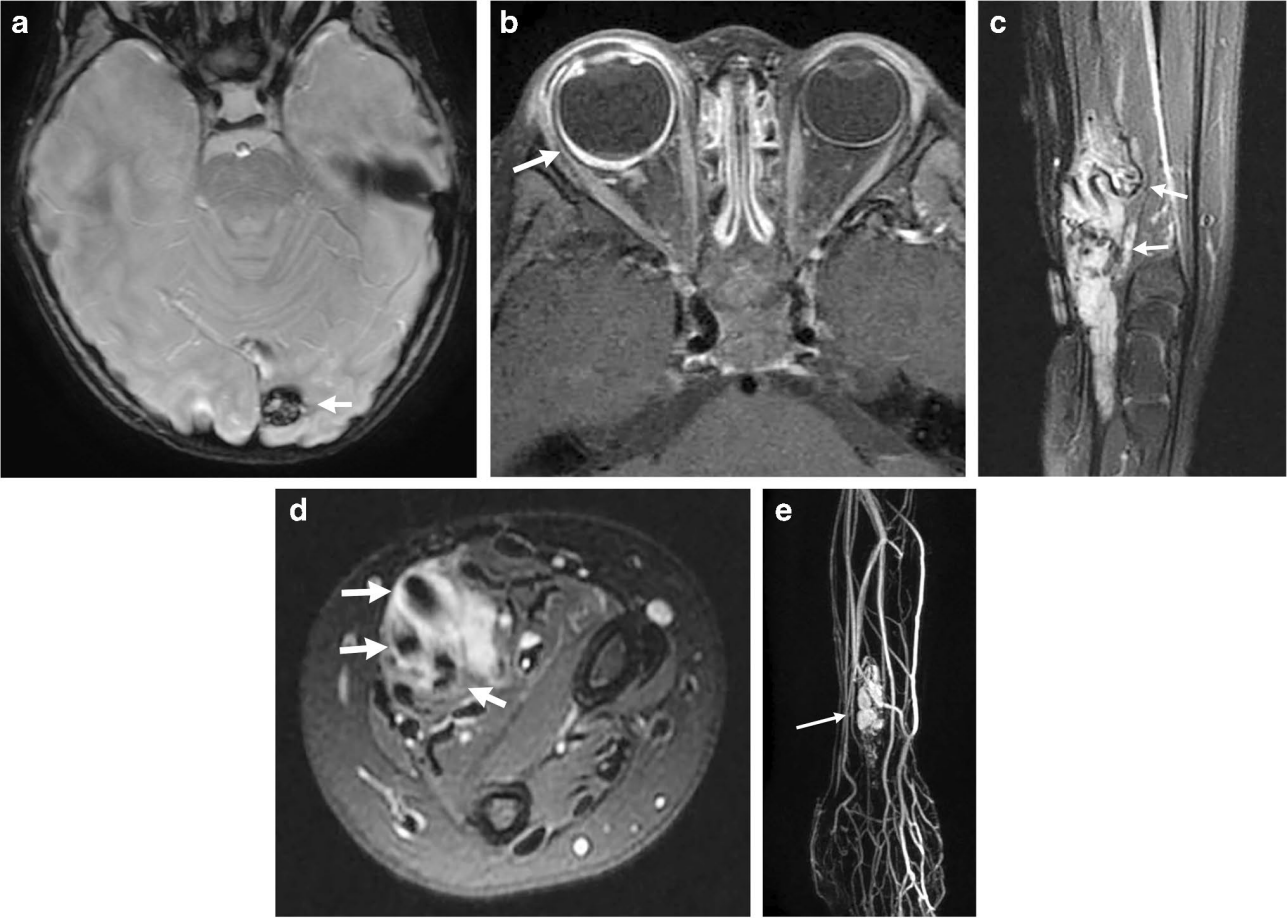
Cowden syndrome in a 3-year-old girl with a genetically confirmed *PTEN* mutation who presented with autism, macrocephaly and a vascular malformation. a An axial susceptibility weighted image shows a left occipital cavernous malformation (*arrow*). b An axial contrast-enhanced fat-suppressed T1-weighted image of the orbits shows an enlarged right-side globe with abnormal choroidal enhancement (*arrow*) consistent with uveal ganglioneuroma after biopsy and surgical removal. c, d Sagittal and axial fat-suppressed T2-weighted images show a predominantly hyperintense intramuscular lesion in the distal forearm and wrist with multiple flow voids (*arrows*). e A coronal contrast-enhanced time-resolved magnetic resonance angiogram/magnetic resonance venogram shows arterial enhancement within the lesion (*arrow*), but there are no large draining veins to suggest an arteriovenous malformation. This mass represents a *PTEN* hamartoma of soft-tissue (PHOST) lesion
